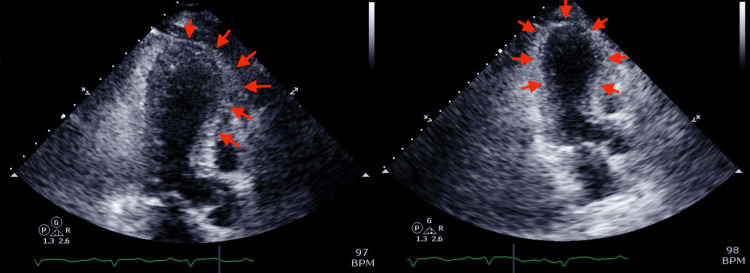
Echocardiogram on admission Left ventricular apical ballooning (depicted by red arrows) is suggestive of takotsubo cardiomyopathy.
